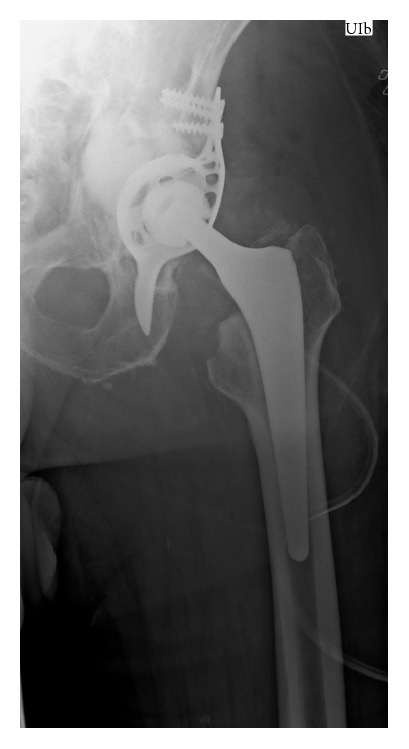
Radiograph of left hip a.p. (supine) on the second day postoperatively.
